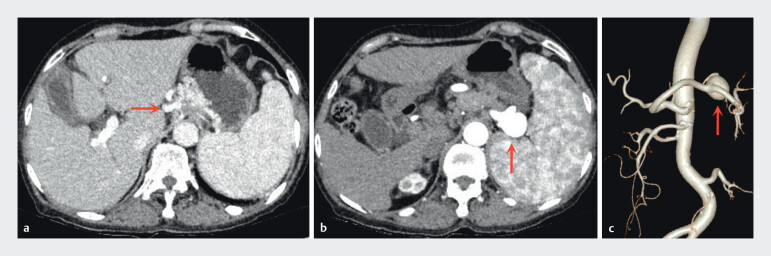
Contrast-enhanced CT images showing
